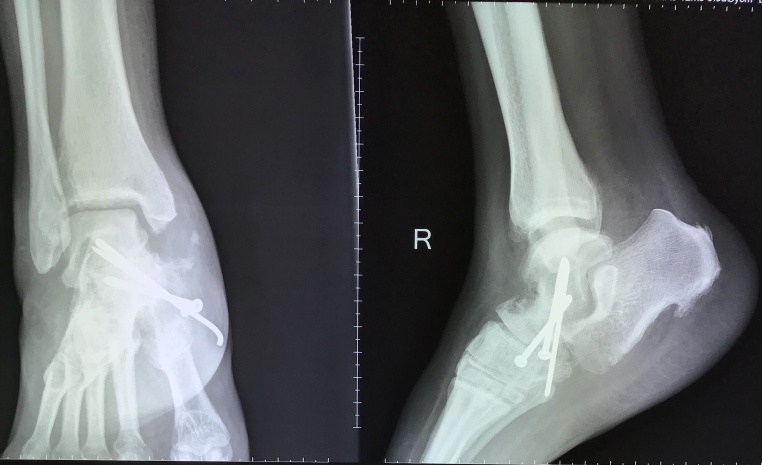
Plain radiograph in three months after forefoot arthrodesis showed the bony union of the talus bone fixed by screws and K-wire.
